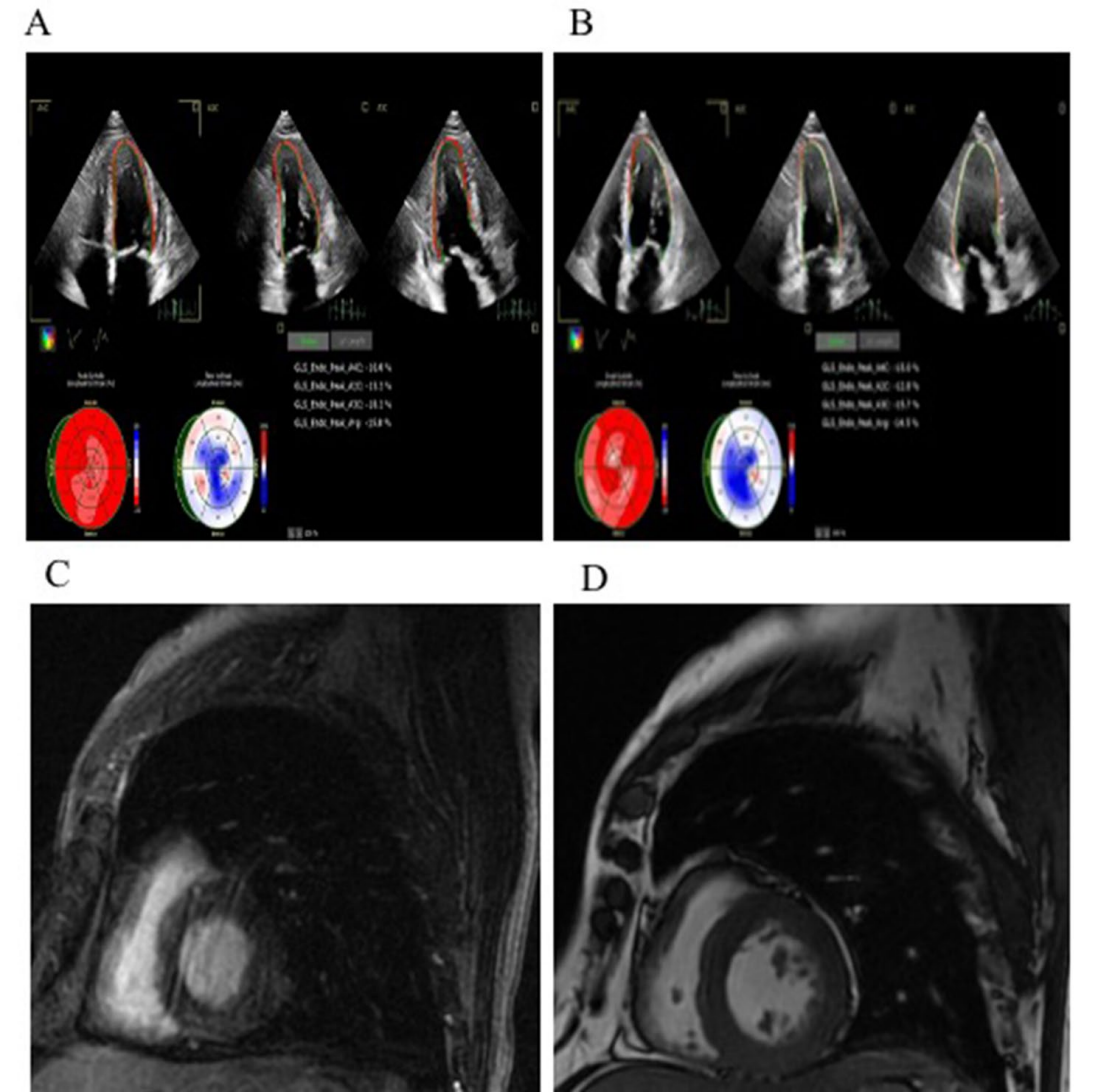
The cardiac ultrasound and cardiovascular magnetic resonance (CMR) in the patient with immune checkpoint inhibitor-associated myocarditis. (A) Cardiac ultrasound demonstrated the LV global longitudinal strain (GLS) was − 15.8%. (B) 20 days after treatment, the LV GLS improved to -14.5%. CMR imaging showed the patient without significantly late gadolinium enhancement (C) and T2 signal intensity (D)
